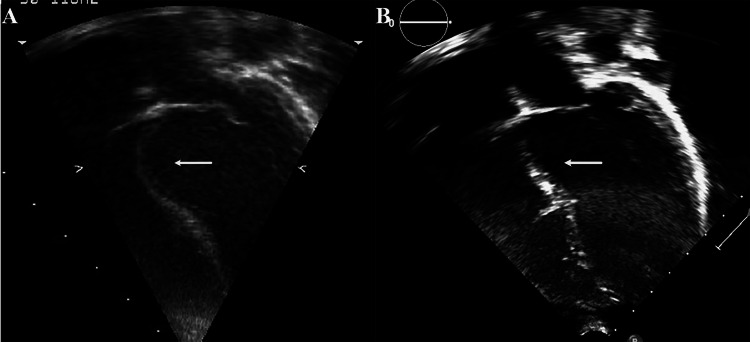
Echocardiogram images at presentation and last follow-up. A. Four-chamber view at end-diastole of the first echocardiogram at 18 months of age. There is an aneurysm of the basal interventricular septum at the level of the atrioventricular valves. Echogenic areas in the interventricular septum suggestive of ischemia/infarction secondary to an embolic event to the coronary arteries. B. Four-chamber view at end-diastole of the echocardiogram at last follow-up (12 years of age). The aneurysm is much less noticeable.
